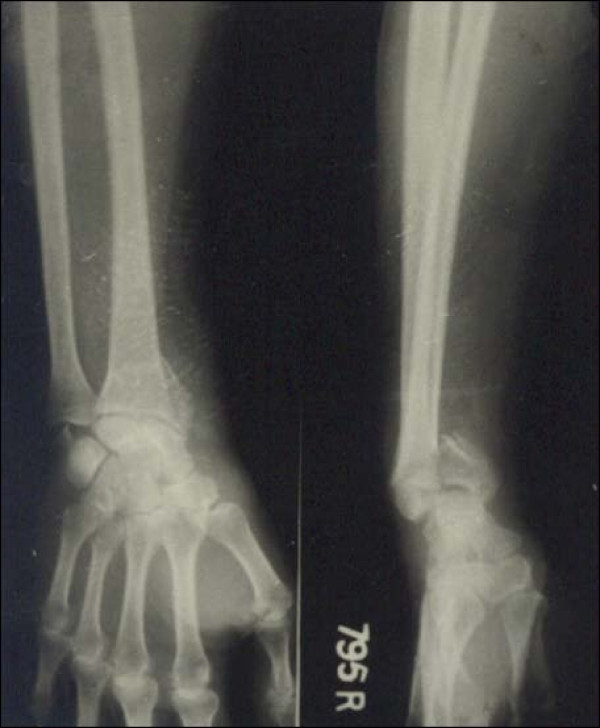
Case 3 Preoperative Radiograph Anteropsterior and Lateral view.
